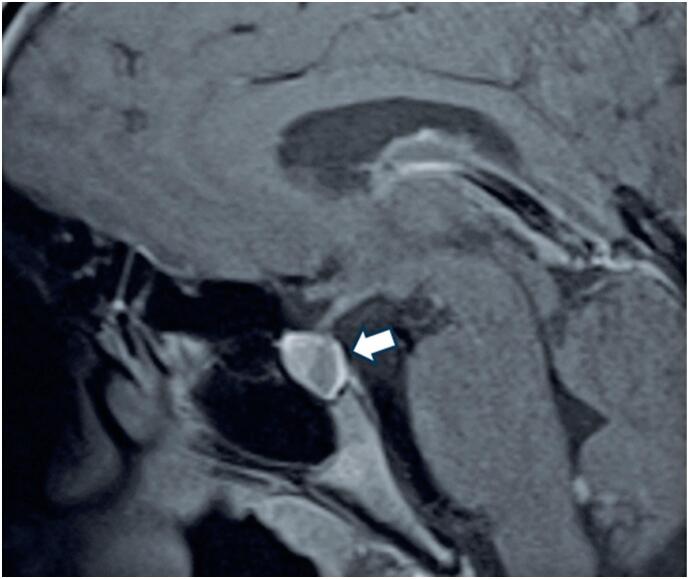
Sagittal post-contrast T1-weighted magnetic resonance imaging showing a non-enhancing, well-circumscribed cystic lesion within the sellar region, consistent with a Rathke's cleft cyst (white arrow). The lesion demonstrates a hyperintense rim with hypointense center, and there is no evidence of suprasellar extension or optic chiasm compression
